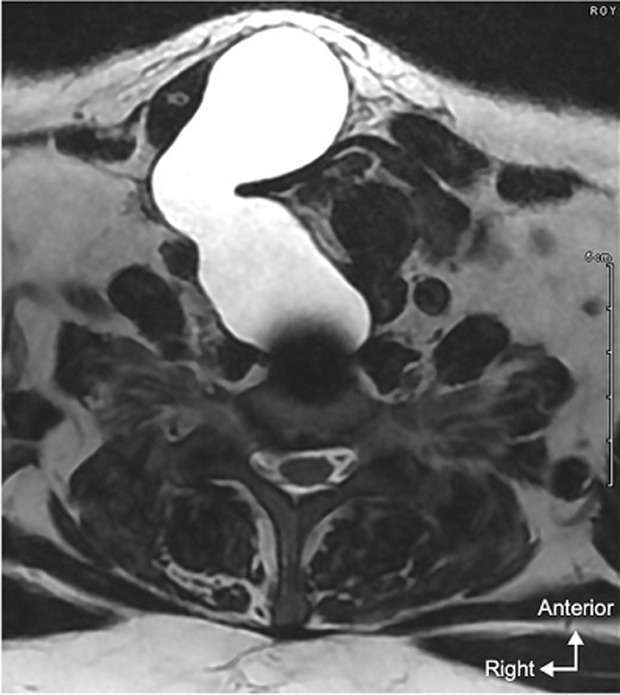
axial T2-weighted MRI showing anterior meningocele with left-displaced esophageal structures and skin fistula
